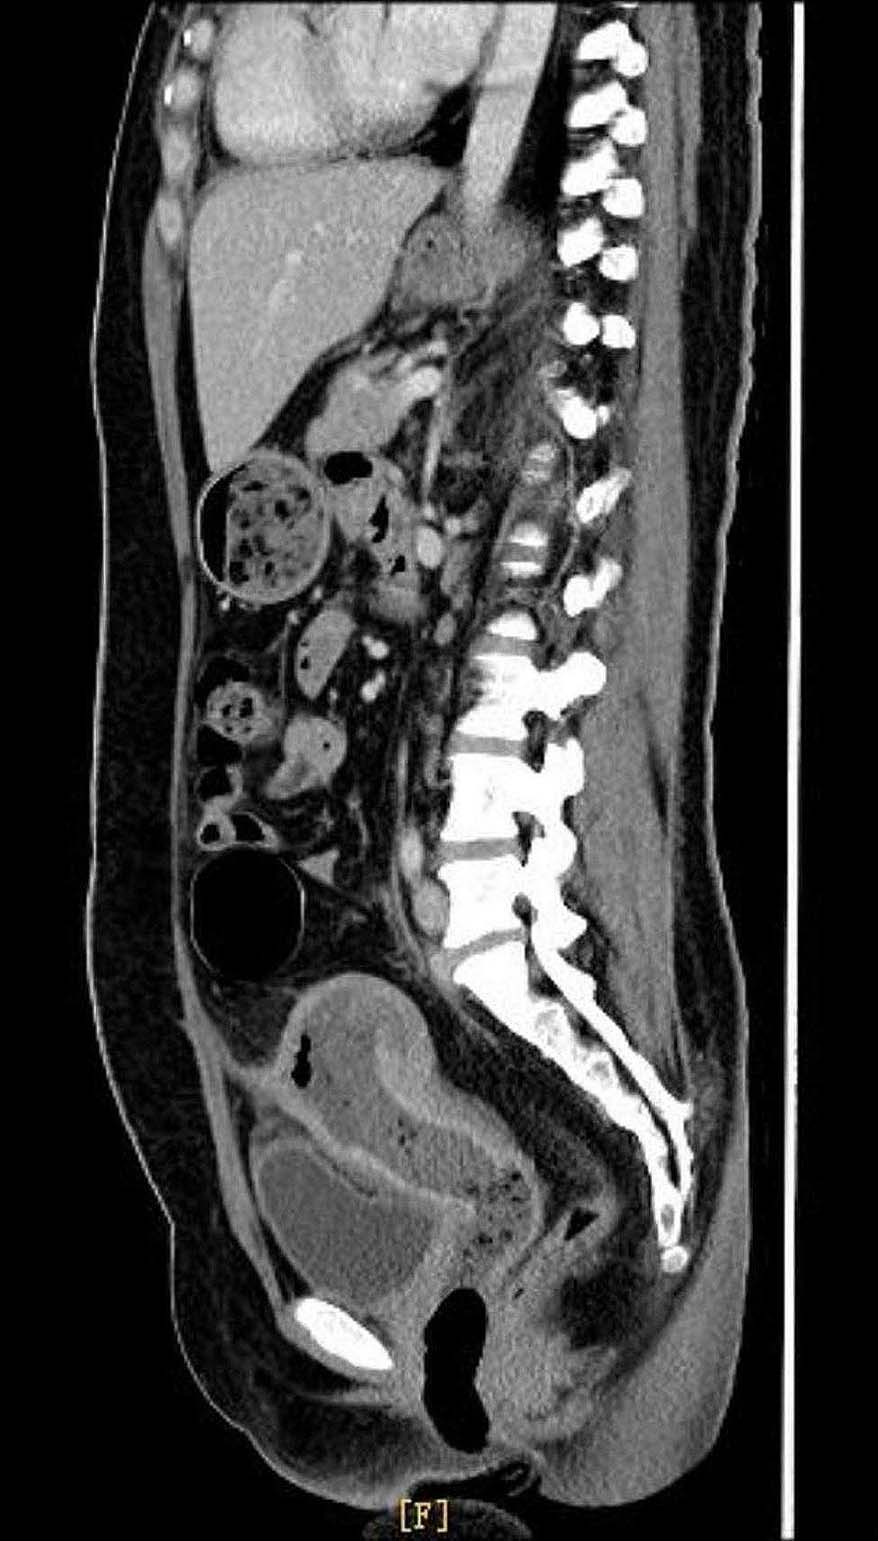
Sagittal computed tomography images. The uterus shows heterogeneous spongiform enlargement with multiple air locules, measuring 4 × 2.6 cm and extending over 5 cm. We have observed a difficulty in distinguishing between the myometrium and the endometrial cavity
